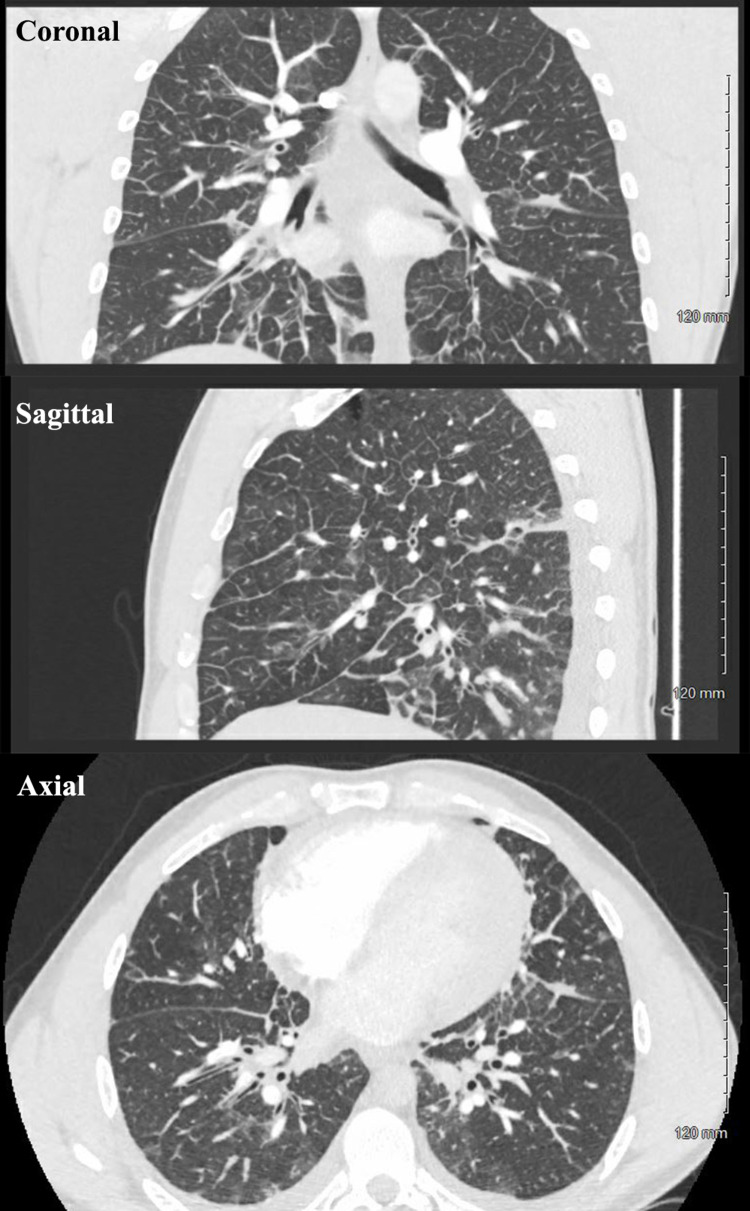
Computed Tomography (CT) Angiography on admission (Hospital day 1) revealing interlobular septal thickening, patchy multifocal ground-glass opacities, small bilateral pleural effusions, and multiple small mediastinal lymph nodes.
